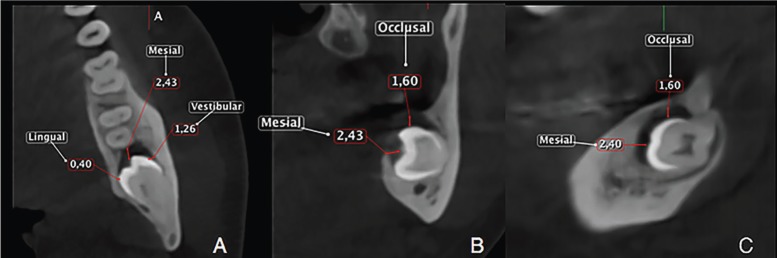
FS measurements performed on different CBCT sections. A. Axial view showing vestibular, mesial and lingual FS measurements on a horizontal impacted third molar B. Vestibular, occlusal and lingual FS measurements performed on a coronal view C. Sagittal view of the FS occlusal measurement.
